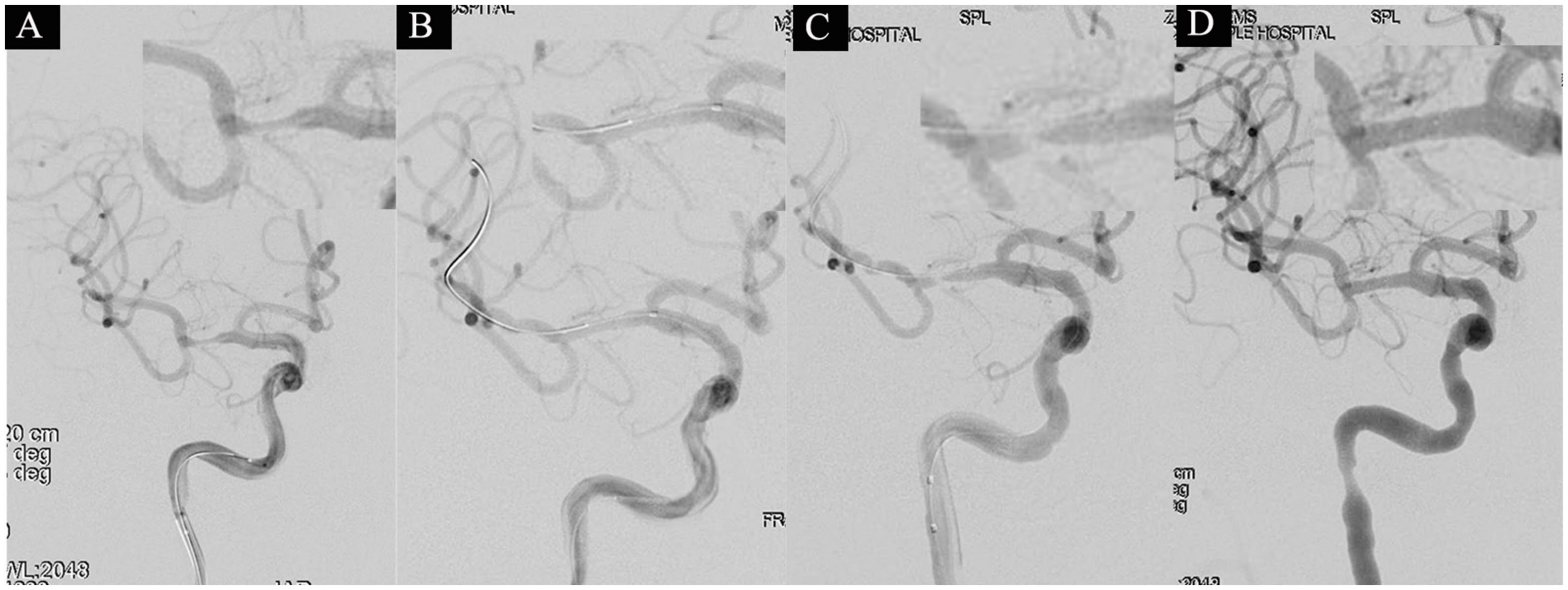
A 53-year-old male with a 3-year history of diabetes was hospitalized on July 3, 2021, presenting with a 2-day history of left-sided limb weakness and numbness. His NIHSS score at admission was 2 points (facial palsy 1 point, dysarthria 1 point). Diagnostic head MRI identified an acute infarction in the right cerebral hemisphere. On July 19 (after 18 days of stroke onset), the patient underwent a balloon angioplasty under general anesthesia to address severe stenosis in the M1 segment of the right MCA. The proximal lumen of the stenosis measured 2.5 mm, with the narrowest part at 0.5 mm, indicating an 80% stenosis degree (A). Following consecutive use of 2.0 × 15 mm and 2.5 × 10 mm balloons, a subsequent angiogram exhibited noticeable improvement in the stenosis (B). However, after a 10-min observation period, there was observed restenosis at the stenotic site (C). Subsequently, a 2.5 × 10 mm balloon-mounted stent (APOLLP, MicroPort, China) was deployed, effectively relieving the stenosis and achieving an eTICI grade of 3 (D).
